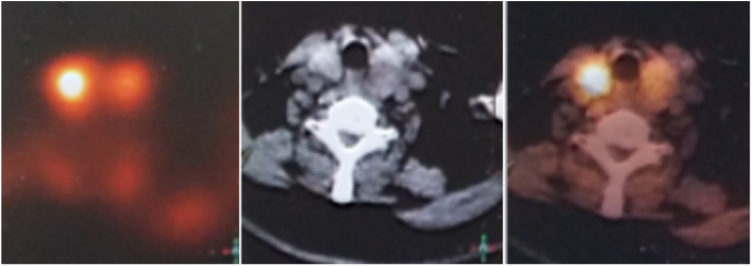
Single-photon emission computed tomography scans revealed a left-side parathyroid gland adenoma.
